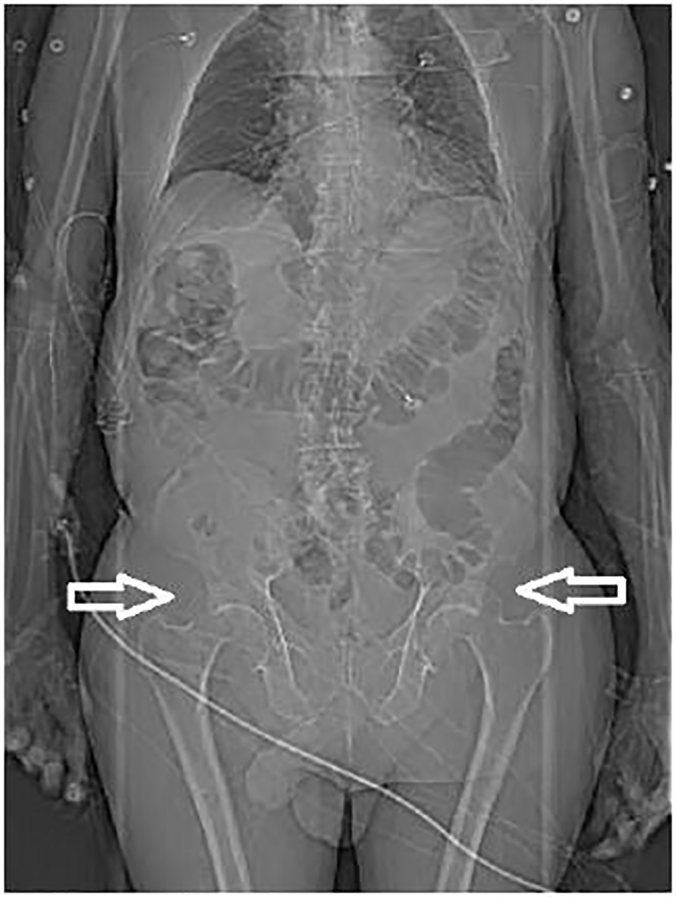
Computed tomography scout image demonstrating bilateral posterior hip dislocations (arrows).
